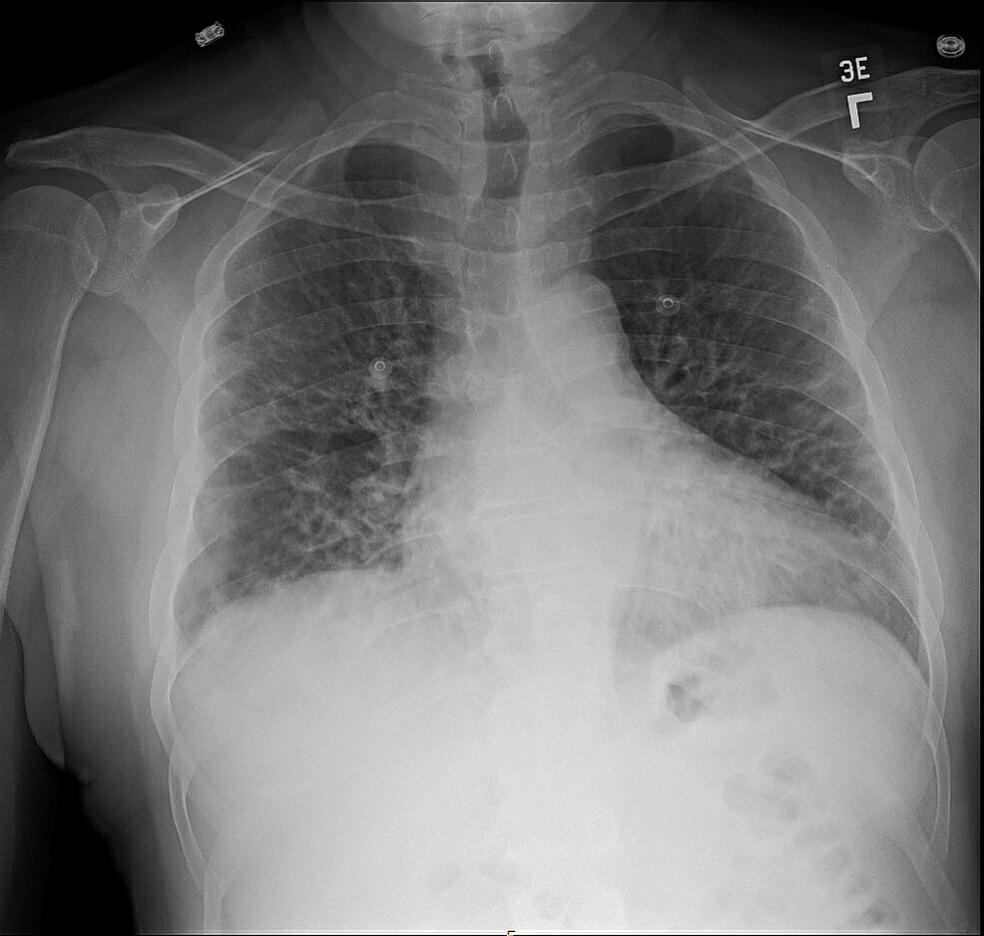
Chest X-ray at time of presentation Chest X-ray showing a normal cardiomediastinal silhouette with evidence of bilateral peripheral hazy opacities. No pleural effusion or pneumothorax is seen.
